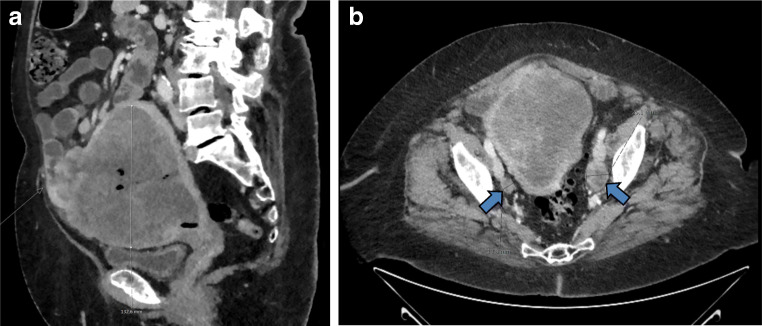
Radiology. a Sagittal CT showing a large heterogeneous tumor filling the uterine cavity, with ventral invasion of the uterine wall and adjacent small intestine loop. Black areas in the tumor represent air, result of biopsy or intestinal perforation, b Axial CT of the pelvis showing enlarged lymph nodes (arrows)
